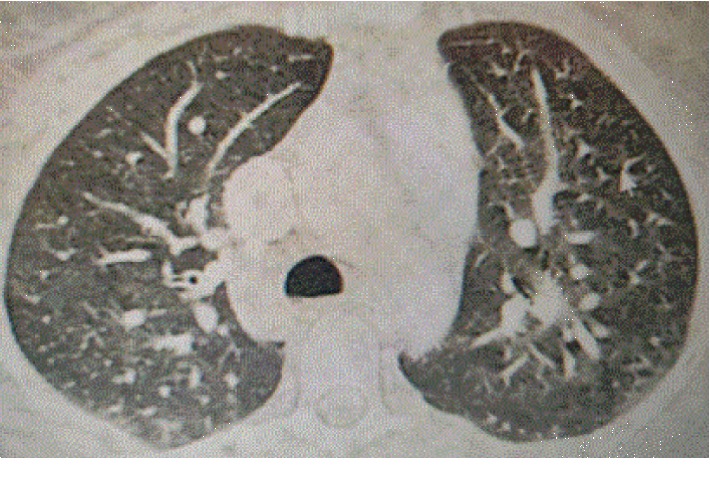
The transversal section of the CT scan shows arteriovenous pulmonary disorders.
